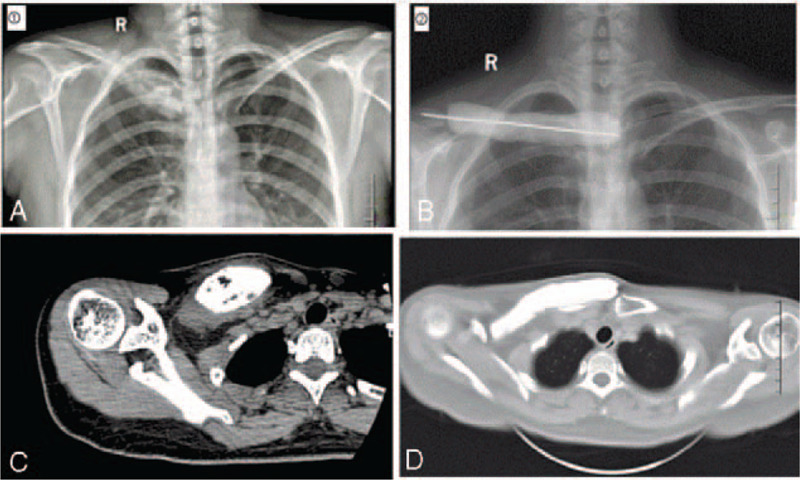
The X-ray and CT films of the patient: (1) at first admission; (2) after spacer implanted.
